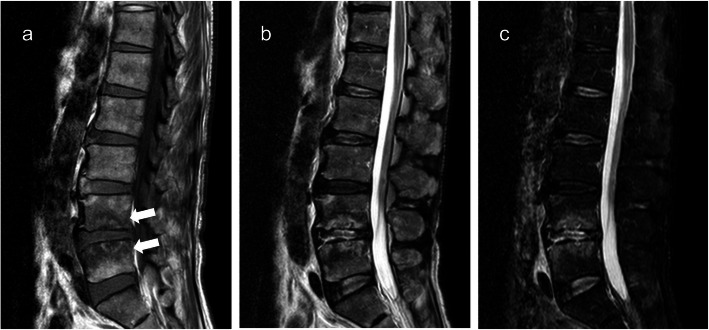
Magnetic resonance images. T1-weighted image (a), T2-weighted image (b), and STIR image (c) revealed pathological changes in the L4 and L5. The signal intensity in the diseased area was low on the T1-weighted image (a, arrowheads) and high on the T2-weighted and STIR images (b, c)
